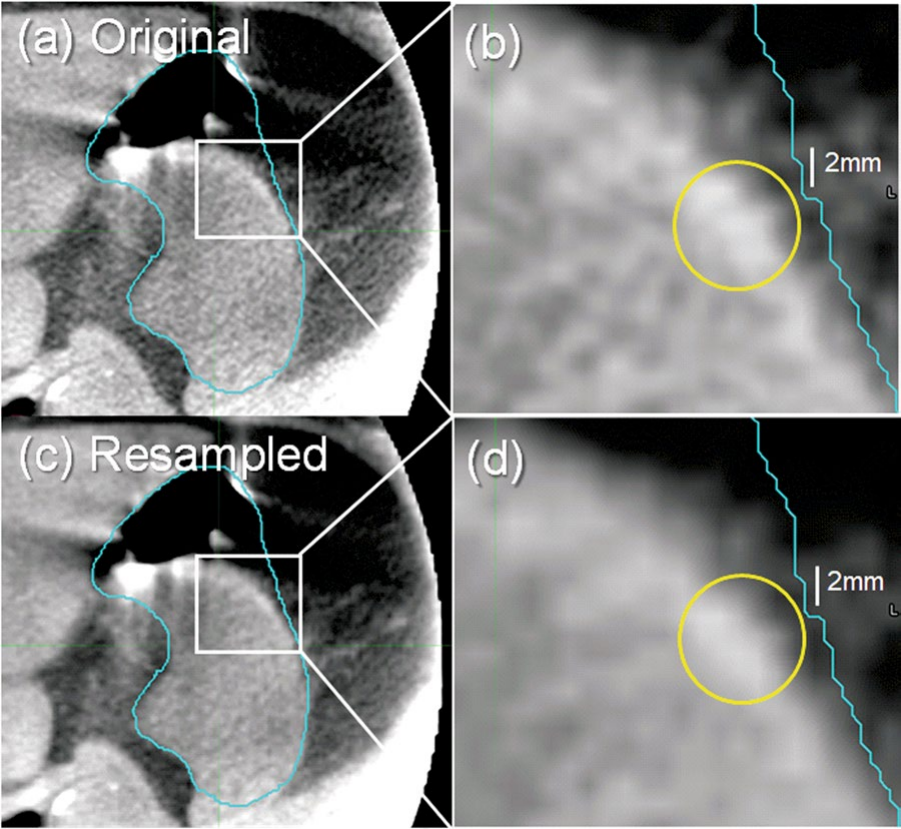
Example of a limited-view CBCT axial slice (a) in original image format, (b) magnified portion of original image, (c) resampled image, and (d) magnified portion of resampled image. Stomach is outlined in all panels. Circles in panels (b) and (d) indicate areas where pixel-scale artifacts are visible.
